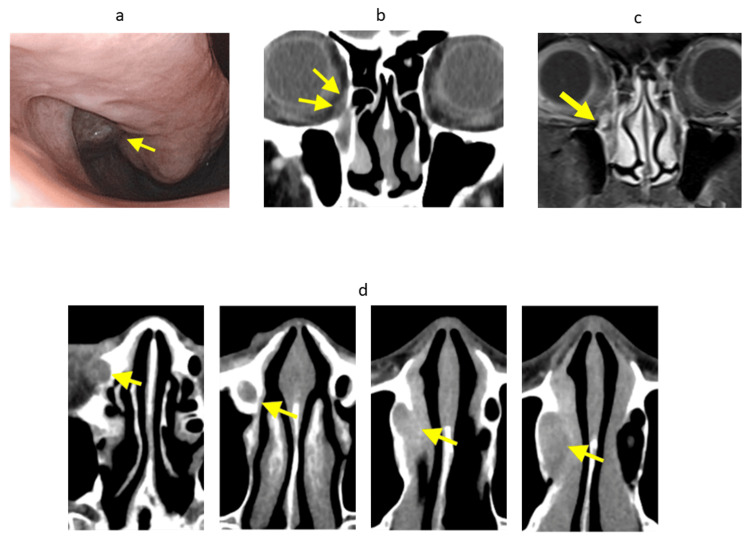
Preoperative endoscopic, CT, and MRI findings showing tumor extension from the inferior nasal meatus to the lacrimal sac (a) nasal endoscope, (b) coronal CT scan, (c) coronal MRI, and (d) axial CT scan. The tumor extended from the inferior nasal meatus along the nasolacrimal duct, reaching the lacrimal sac. Arrow: tumor, CT: computed tomography, MRI: magnetic resonance imaging
